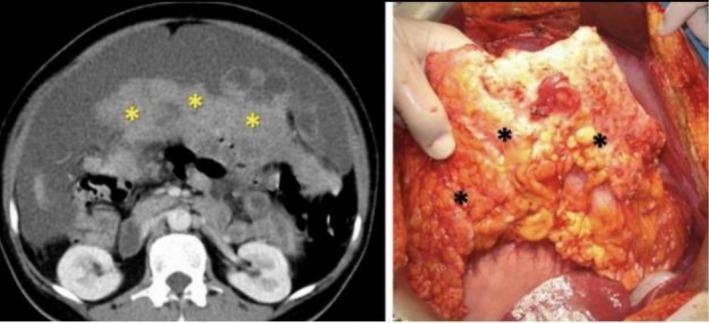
Contrast‐enhanced CT showing peritoneal carcinomatosis with ascites (left) along with the corresponding gross intraoperative appearance.(right).
